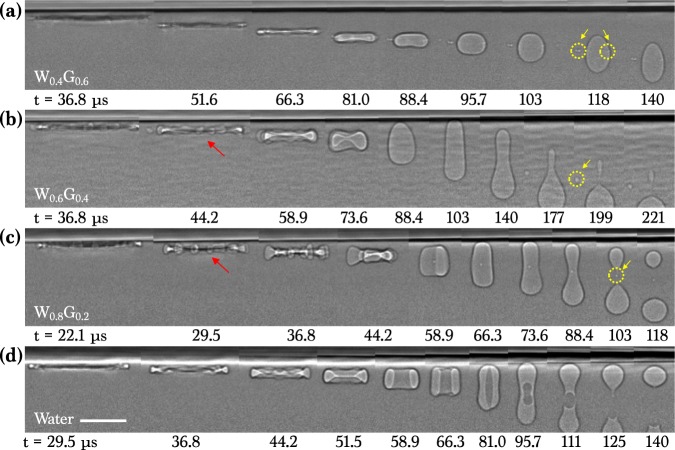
X-ray images of air evolution for water-glycerol mixtures (W-G). Imaging condition is the same with Fig. 2. (a) Impact of a W_0.4_G_0.6_ drop (*μ* = 10.9 mPa s) at *H* = 12 cm. (b) Impact of a W_0.6_G_0.4_ drop (*μ* = 3.7 mPa s) at *H* = 12 cm. (c) Impact of a W_0.8_G_0.2_ drop (*μ* = 1.7 mPa s) at *H* = 12 cm. (d) Impact of a water drop (*μ* = 1.0 mPa s) at *H* = 12 cm. Here, one bubble in (a), a double bubble in (b–d), and additional tiny bubbles (yellow circles) were generated. The scale bar is 100 *μ*m long.
